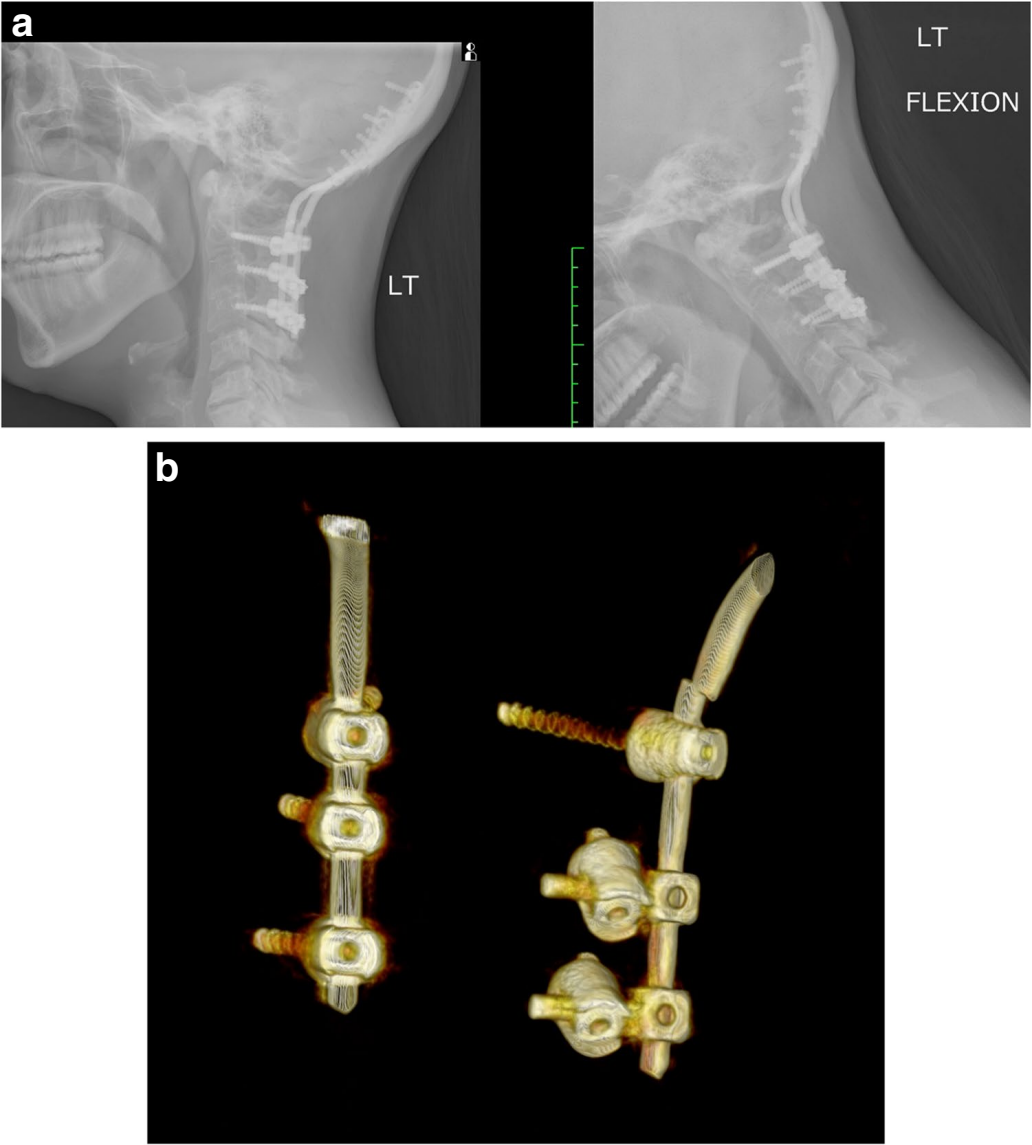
A X-rays cervical spine in flexion and extension. Lateral view of broken traditional barplate hardware. B 3D CT of broken traditional barplate, posterior view
